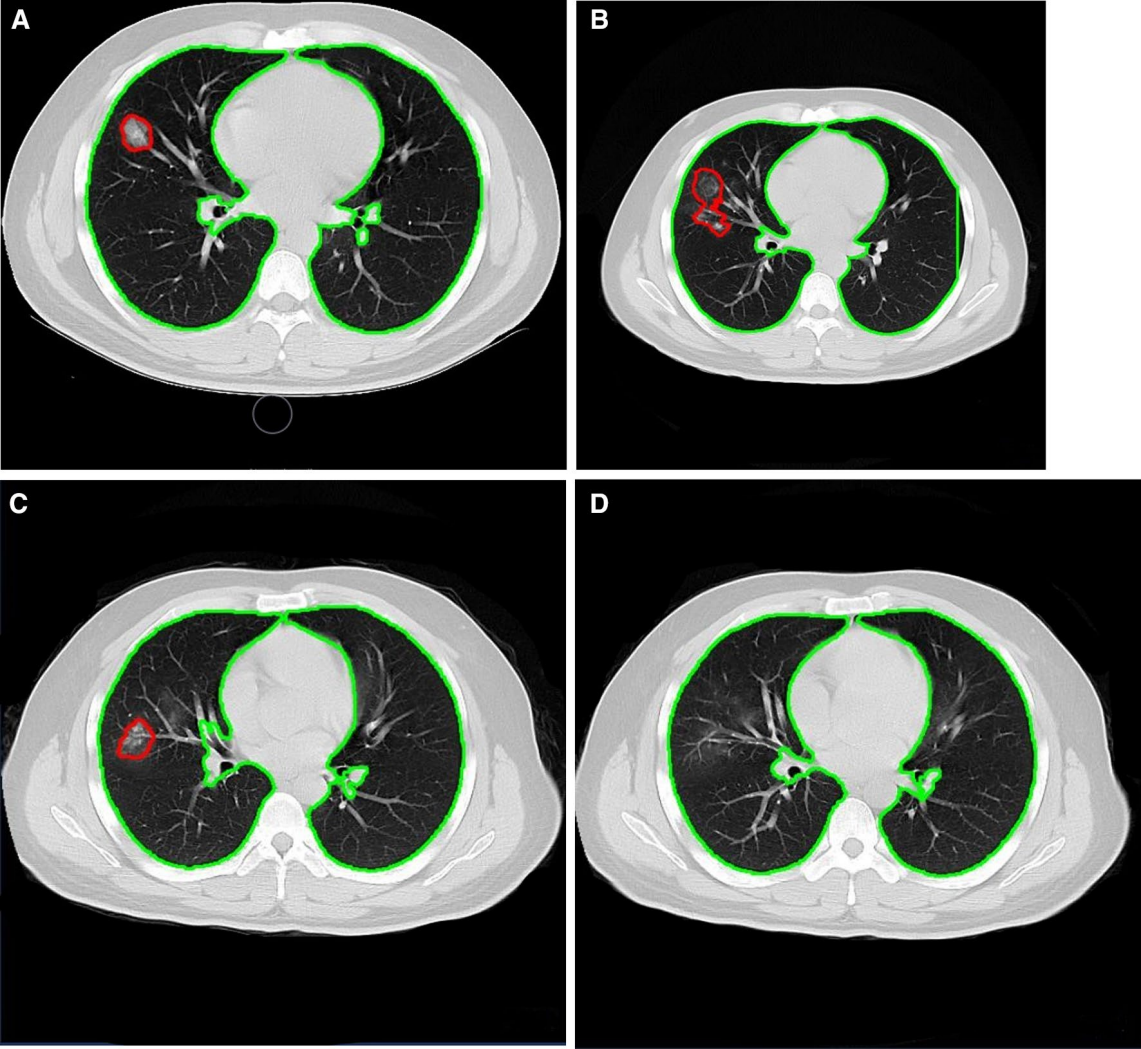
Longitudinal CT in a 29-year-old male with mild COVID-19. A The initial CT examination showed a GGO in the middle lobe of the right lung; B the lesion enlarged, and new GGO appeared (accounting for 1.32% of the right lung volume); C GGO was absorbed compared with the second inspection; D The GGO remained at follow-up 30 days later
